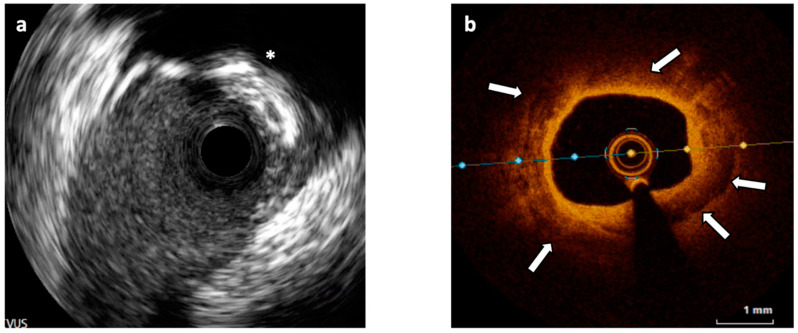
(a) Intravascular Ultrasound (IVUS) cross-section showing a 180° calcified plaque opposite to a side branch origin and producing shadow (asterisk). (b) Optical Coherence Tomography (OCT) cross-section of a different segment of the vessel showing an almost concentric calcified plaque visible as signal-poor regions with sharply delineated borders (white arrows).
